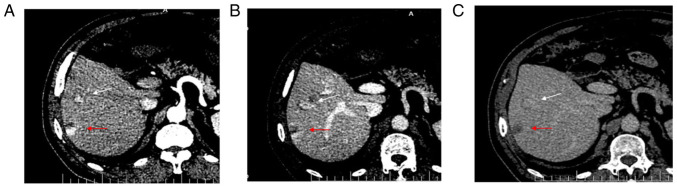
CT of the abdomen. (A) Contrast-enhanced CT showing local enhancement of both the segment 5 (white arrow) and segment 6 (red arrow) lesions in the arterial phase. (B) Enhanced CT showing continuous enhancement of both lesions in the portal vein phase. (C) Enhanced CT showing continued enhancement of the lesion during the delayed period, without significant clearance. Contrast-enhanced CT revealed a benign hepatic haemangioma. CT, computed tomography.
